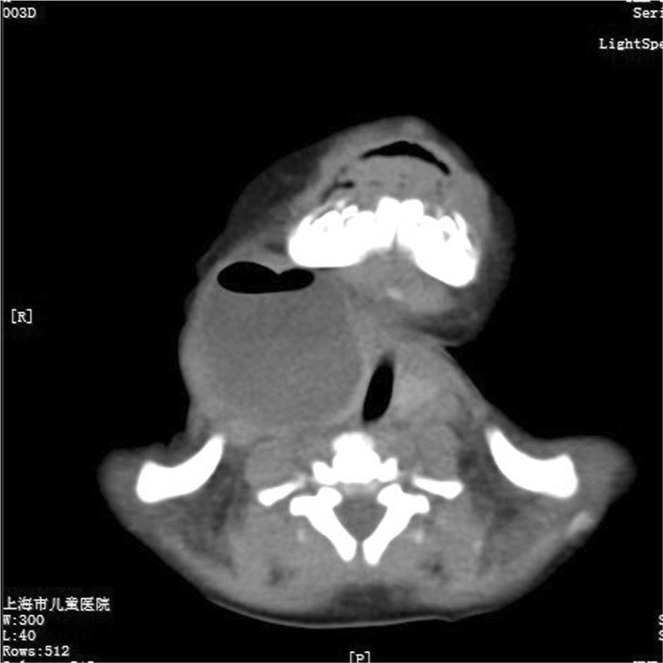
Axial computed tomography scan shows an air-filled cyst with deviation of the trachea (the same patient in Fig. 1).
